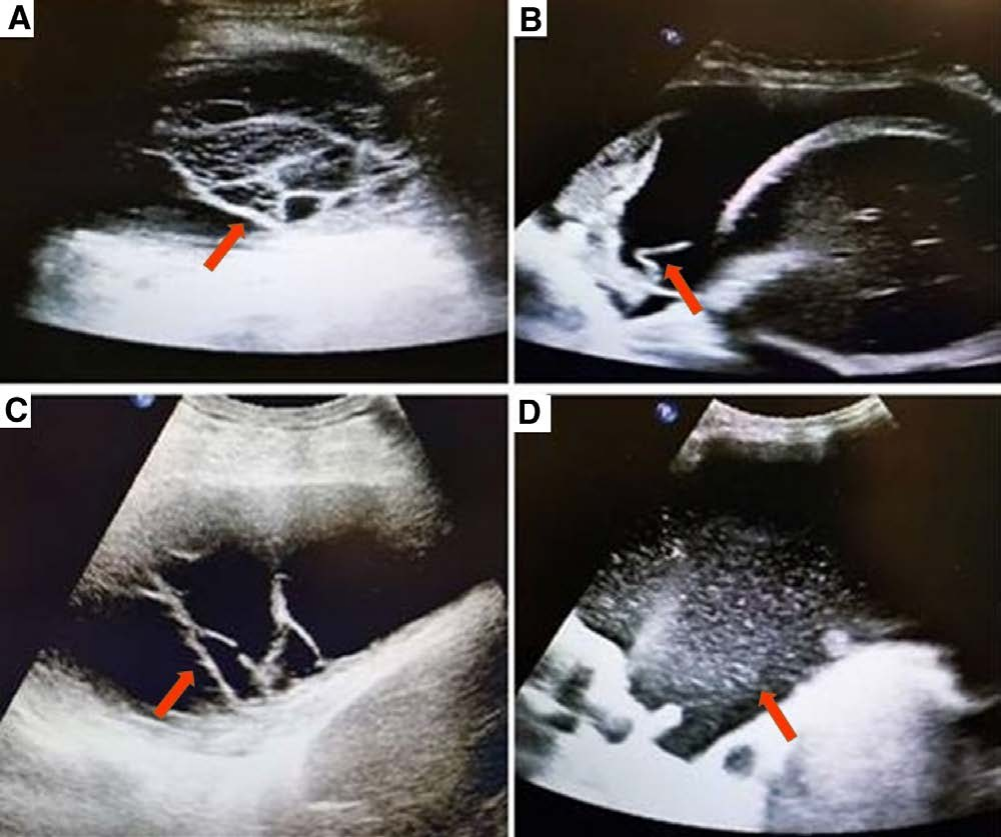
(A) A 44-year-old man with right empyema. TUS showed complex septate appearance and septa were found inside the effusion, as indicated by the red arrow. (B) A 28-year-old woman with left complicated parapneumonic pleural effusion had complex nonseptate appearance in ultrasonographic image. Echogenic material could be seen, as shown by the red arrow, presenting heterogeneously in anechoic space. (C) A 56-year-old woman who was diagnosed as systemic lupus erythematosus (SLE) with left pleural effusion and had complex nonseptate appearance in ultrasonographic image. As indicated by the red arrow, heterogeneous echogenic material could be observed. (D) A 46-year-old man with left empyema. Complex homogenous fluid could be found by the TUS and a large number of light spots could be noted, shown by the red arrow.
